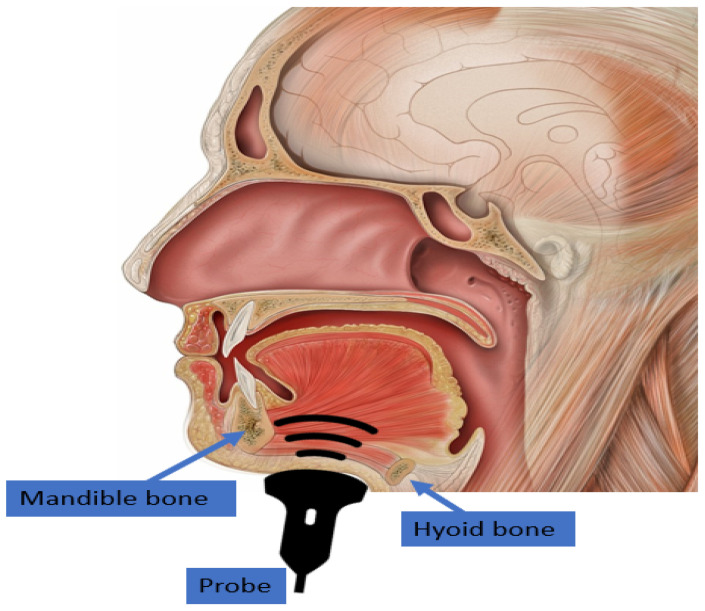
Overview of ultrasound probe placement beneath the chin. The ultrasound wave is shown in a black arc generated from the acoustic probe and propagated in the direction of the tongue. The effect of the hyoid and mandible bones is blocking part of the ultrasound wave, as shown in a black colour. The head and oral cavity picture was modified from the original picture for the case, courtesy of Associate Professor Frank Gaillard, Radiopaedia.org, rID: 35836, [86].
